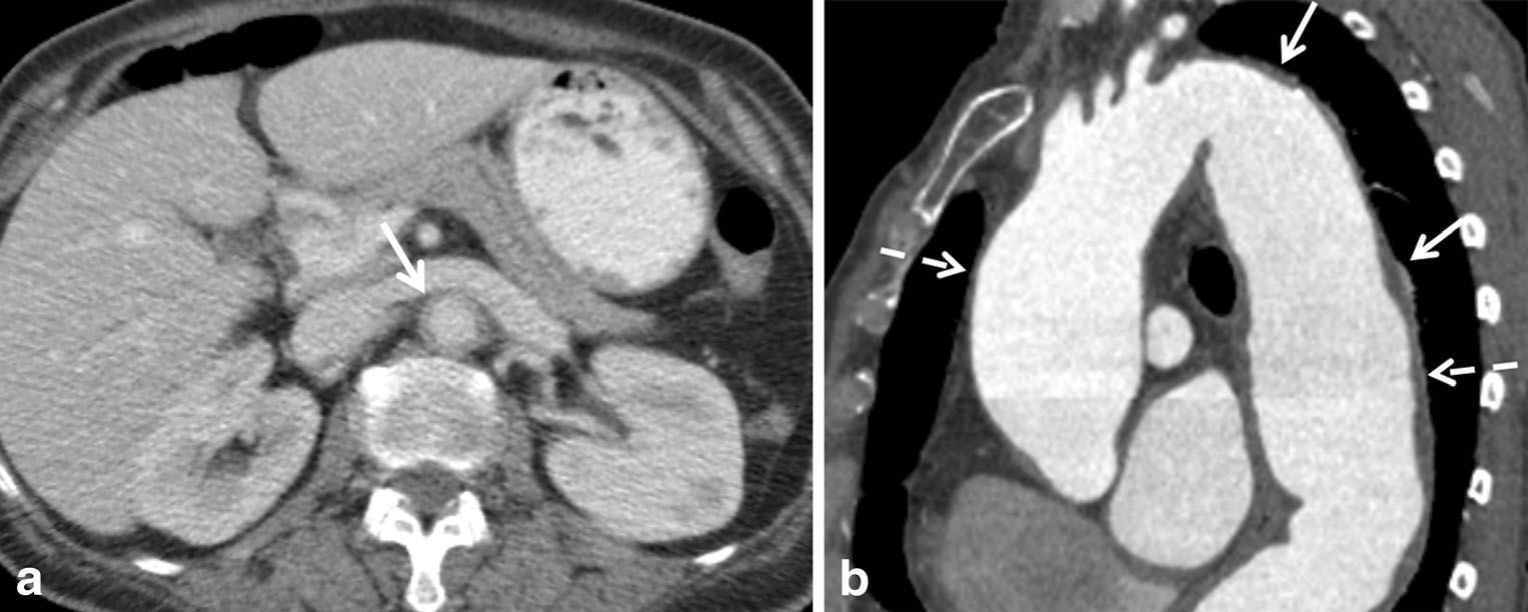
A 58-year-old woman presented with intermittent episodes of atypical, acute chest pain/ epigastric pain and night sweats. In an earlier episode (elsewhere) she had received gastroscopy and a CT scan of the abdomen that showed no abnormalities. At our hospital, blood was drawn that showed anaemia with a haemoglobin level of 5.1 mmol/L. A CT was performed under suspicion of malignancy. (a) Contrast enhanced axial CT shows a thickened aortic wall (arrow), consistent with large vessel vasculitis. Biopsy of the temporal artery proved temporal arteritis, consistent with the diagnosis of giant-cell arteritis. Pericardial effusion was also present (not shown). (b) Sagittal curved reformat of a contrast enhanced CT in a patient with known Takayasu arteritis, showing wall thickening (solid arrows) and an aneurysm of the ascending and descending aorta (dashed arrows)
